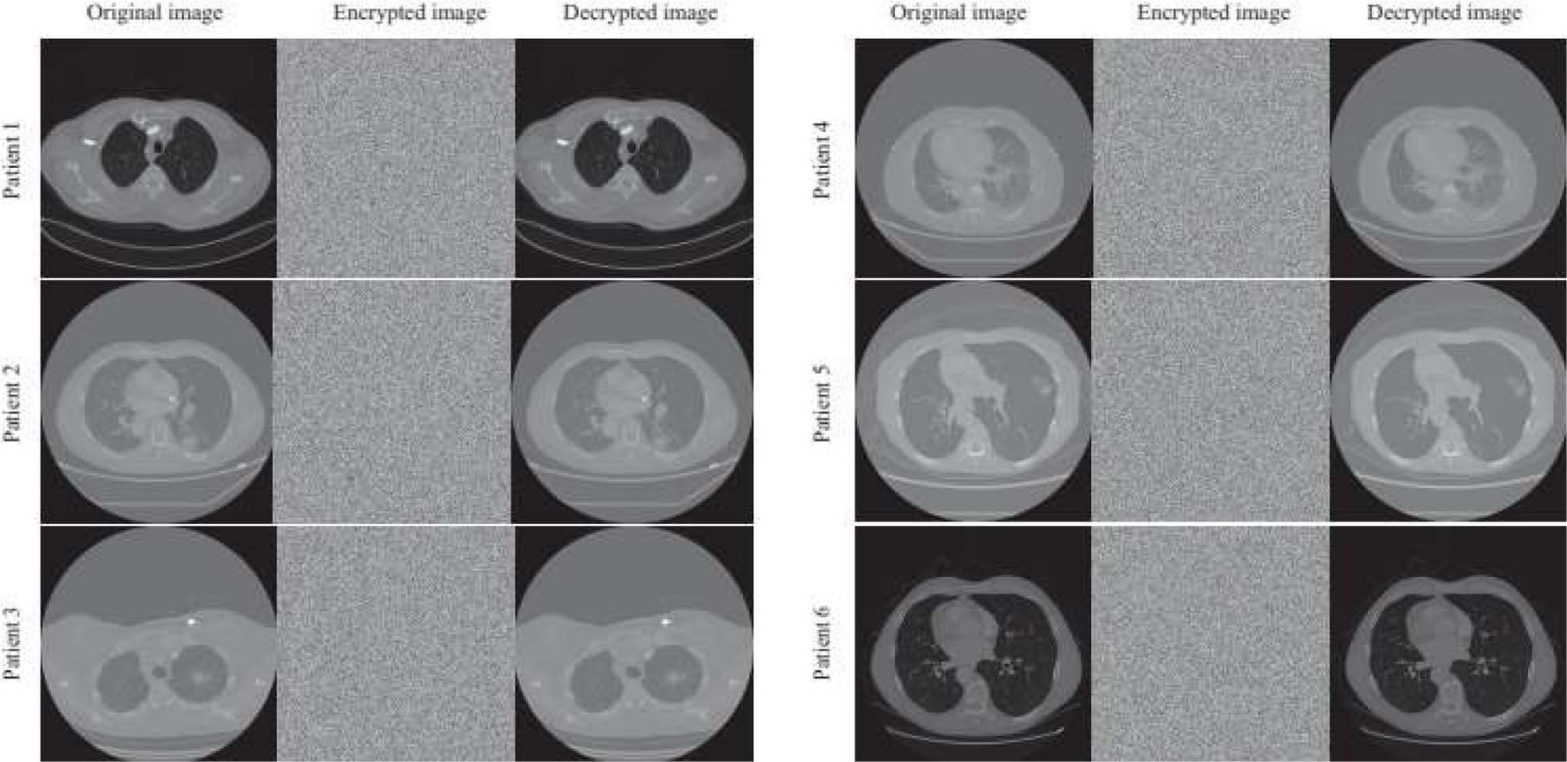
Effect of the AES-CBC encryption algorithm on CT cross-sectional Images of six random patients
